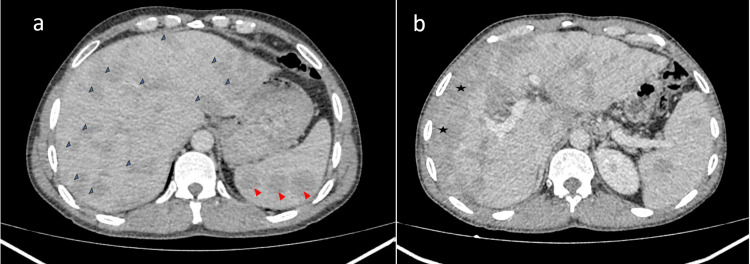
(a) Abdominal CT scan shows an enlarged liver with multiple hypodense nodules (the largest are marked by blue arrows). The spleen contains multiple hypodense nodules (red arrows). (b) Some liver nodules are confluent, forming irregular, ill-defined hypodense areas (stars).
